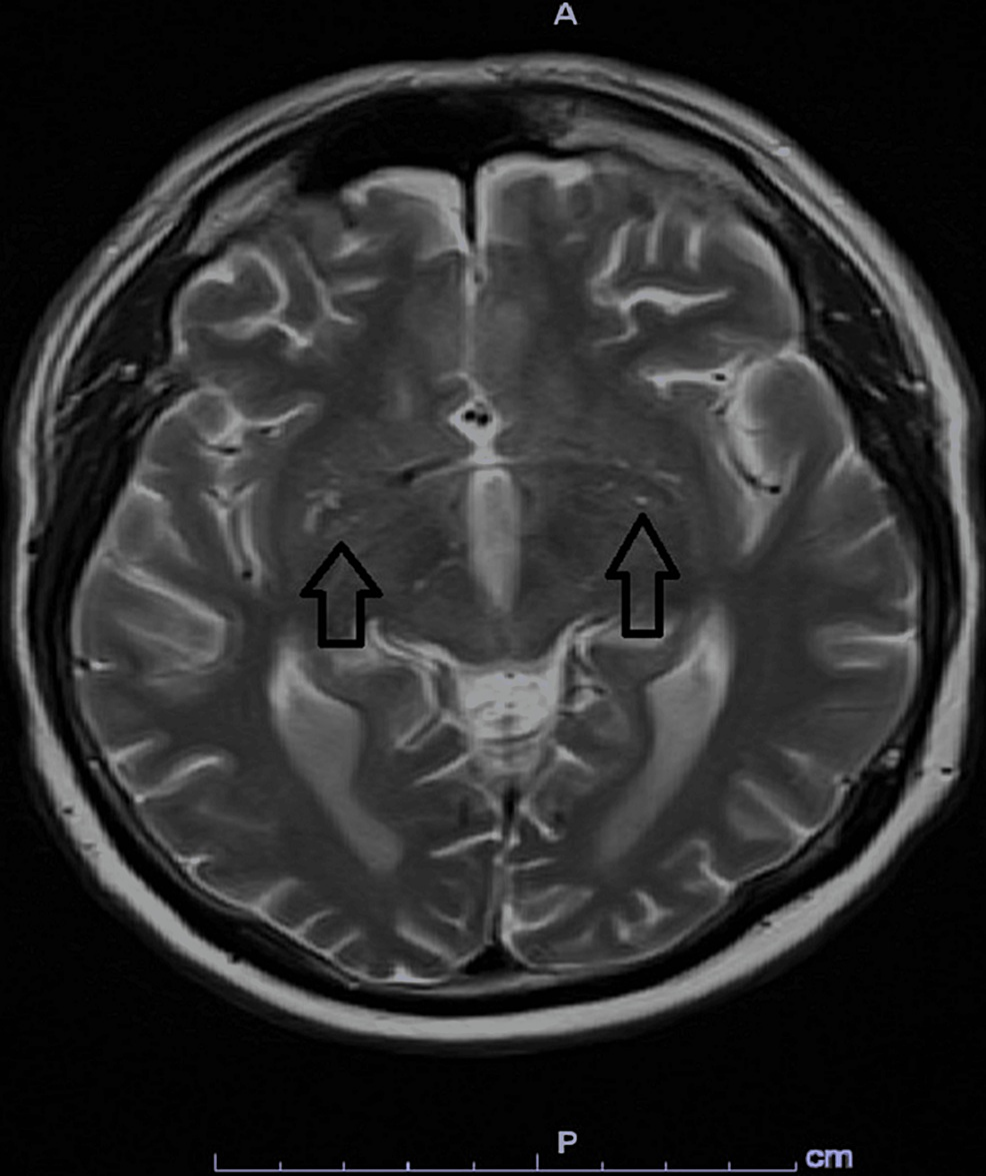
A T2 MRI with no evidence of lesion nor leptomeningeal enhancement.
